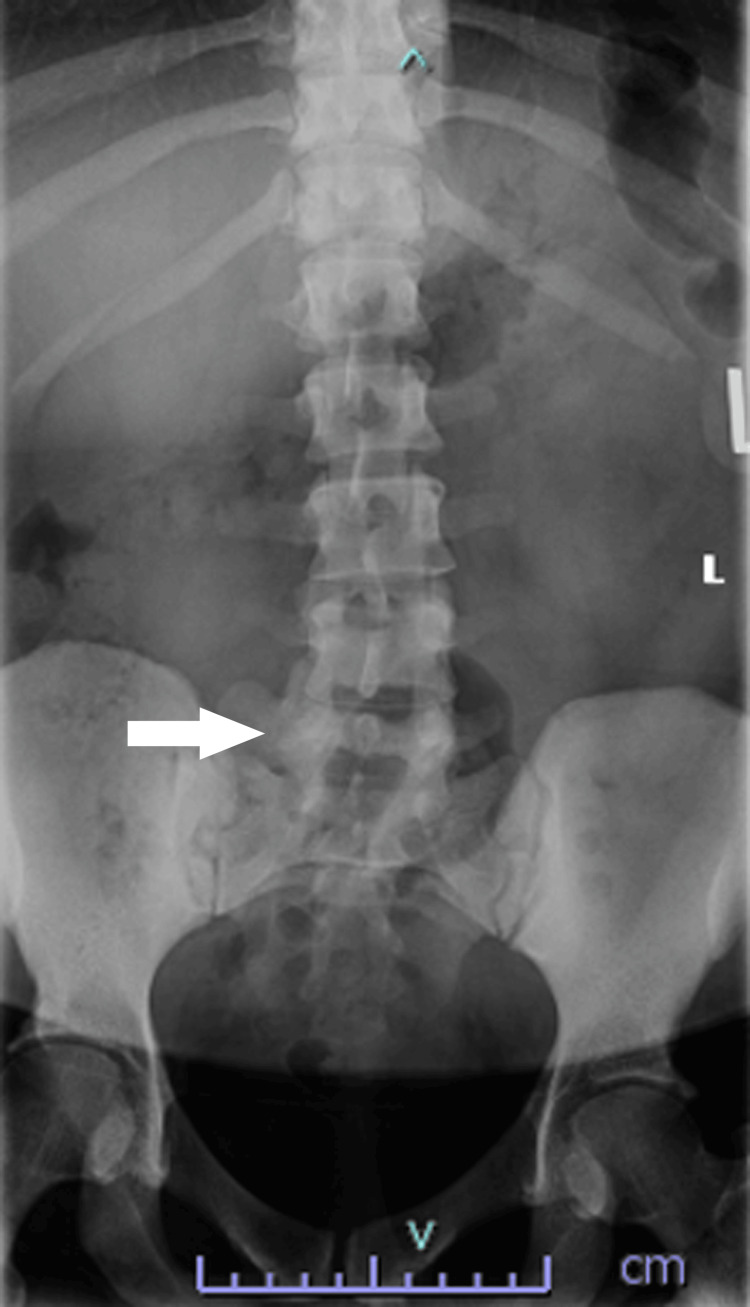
X-ray shows lumbar scoliosis in this patient. The arrow points to the lumbar scoliosis at her L4 level.
